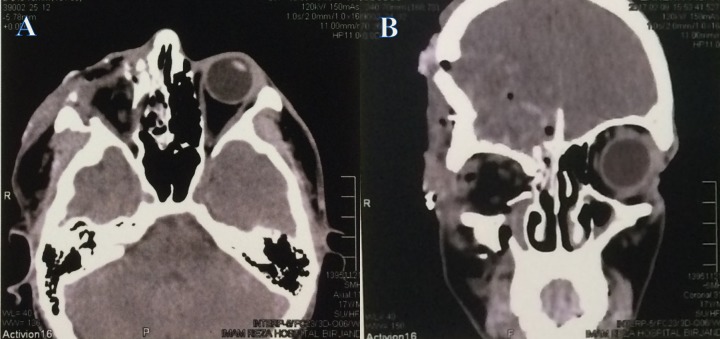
The computed tomography scans. A: An axial view of the orbital CT scans demonstrating the medial and lateral wall of the orbit was fractured. There were no clues that indicate the presence of a globe, B: The coronal view of the orbital CT scans revealed the four walls fracture of the right orbit. The frontal bone is extensively inferiorized. Pneumocephalus and severe adjacent brain injuries were evident. No sign of the right globe was visualized.
